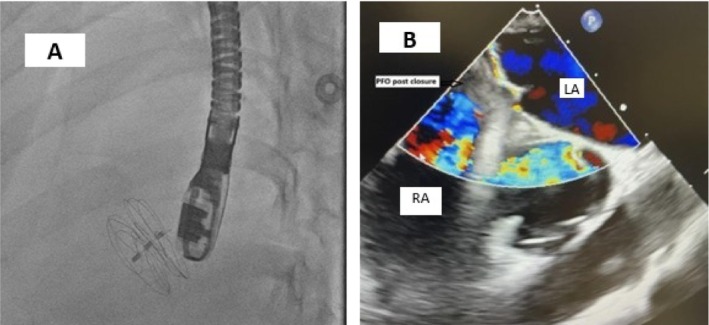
(A) Placement of the Gore septal occluder guided by TEE. (B) Color Doppler TEE in supine position, with a black arrow showing complete resolution of the PFO.
